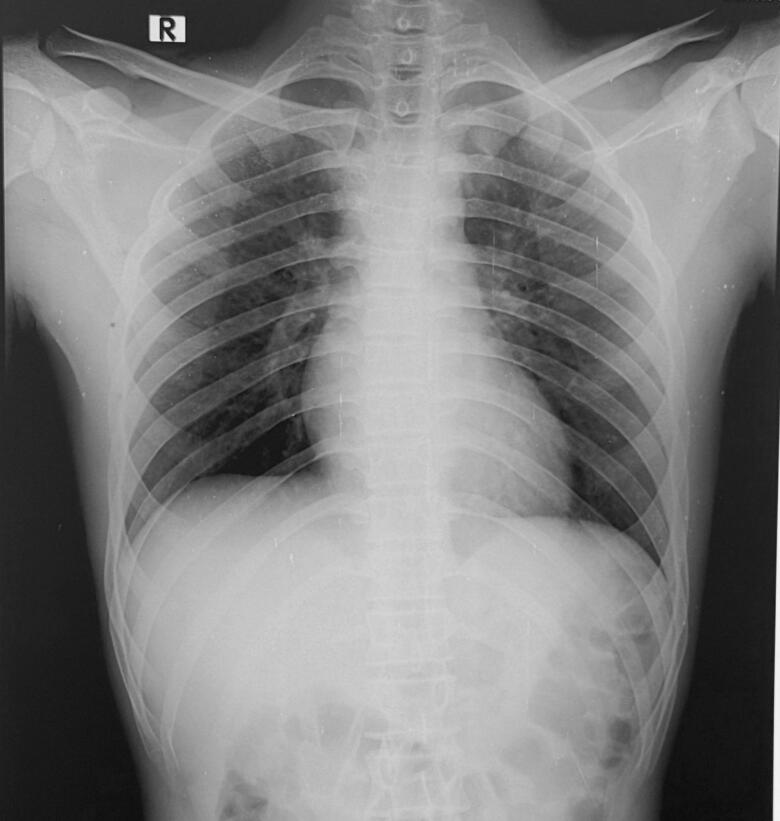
Chest x-ray showing lung fields clear bilaterally with no evidence of ribs fractures or pneumothorax. The cardiac silhouette and mediastinum appear within normal limits.
